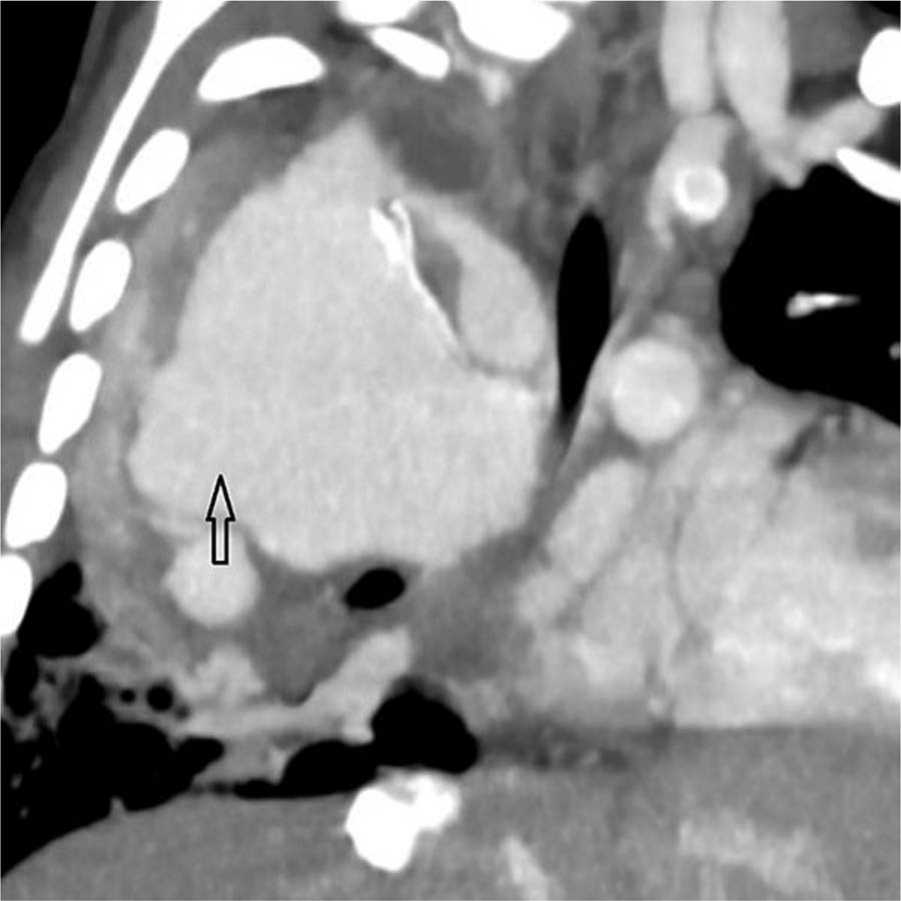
Coronal oblique maximum-intensity-projection reconstruction of thoracic CT angiography showing a large thrombosed pseudoaneurysm of the right subclavian artery at the anastomosis with the modified Blalock–Taussig–Thomas shunt, causing significant mediastinal mass effect.
